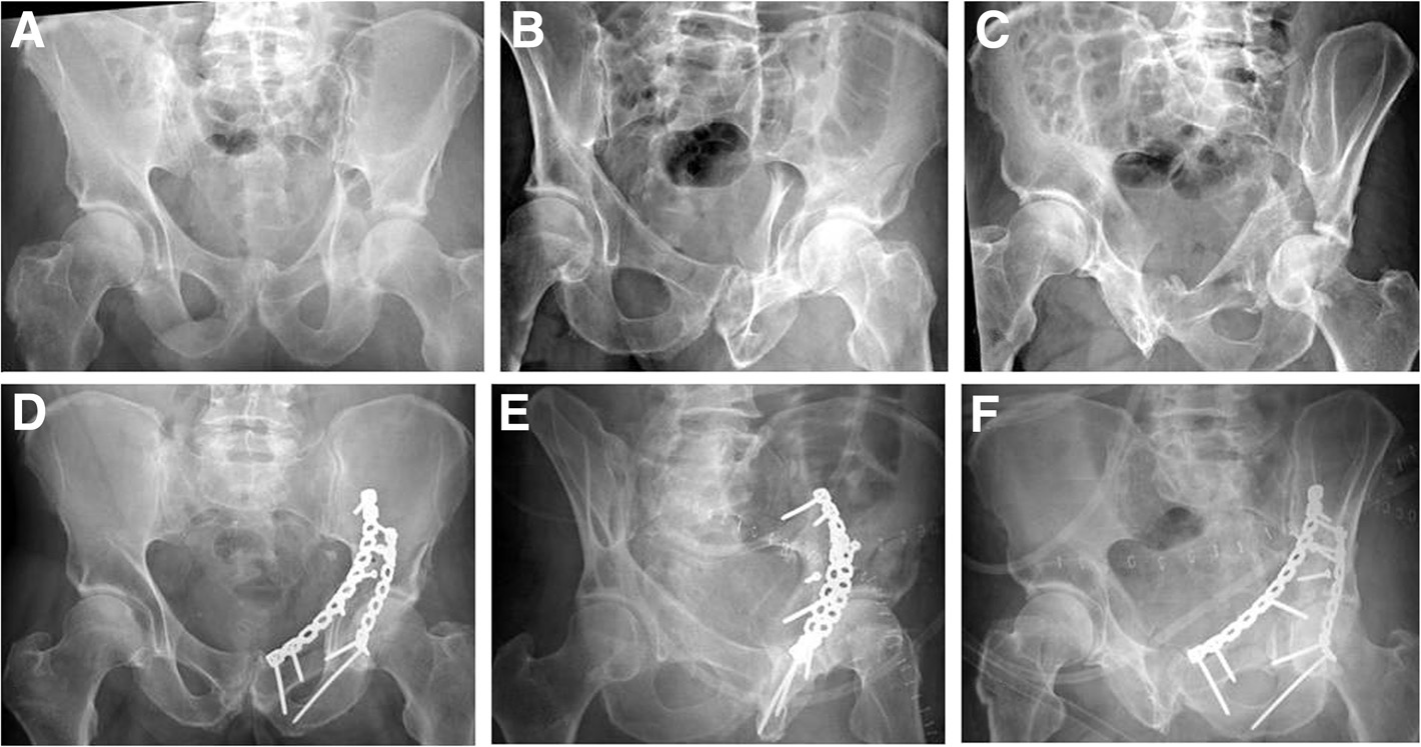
Example of a T-shaped fracture treated via a combined ilioinguinal and Kocher-Langenbeck approach, using two 3.5 mm reconstruction plates and two 3.5 mm lag screws. (A-C) Preoperative X-rays (a.p., obturator oblique, iliac oblique). (D-F) Postoperative X-rays (a.p., obturator oblique, iliac oblique).
